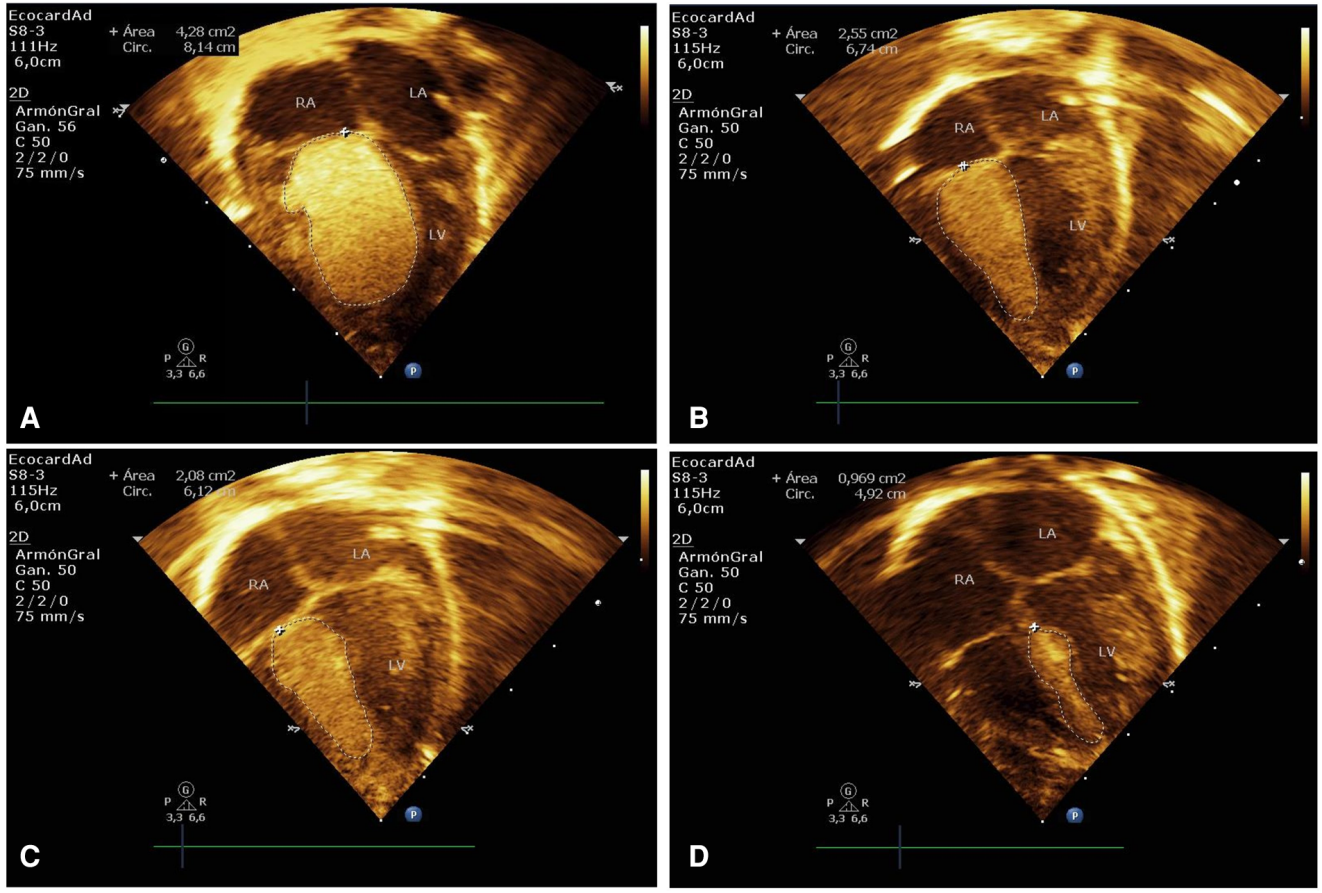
Case 2. (A) Immediate postnatal transthoracic echocardiogram in apical 4-chamber projection showing a giant rhabdomyoma measuring 30 mm × 17.3 mm (area of 4.4 cm^2^), adhered to the interventricular septum, and occupying a large part of the right ventricular cavity. (B–D) Echocardiographic control at 2, 4, and 11 weeks of treatment with everolimus, showed a significant reduction in the size of the rhabdomyoma.
